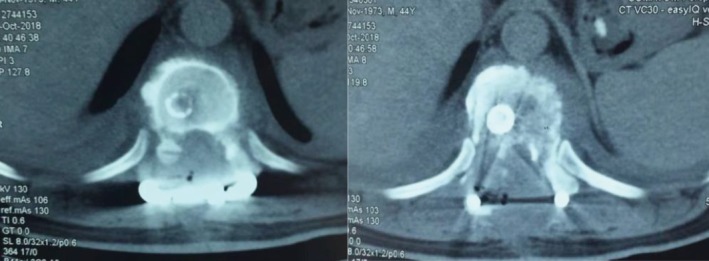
CT Scan before SBRT precision radiotherapy.
